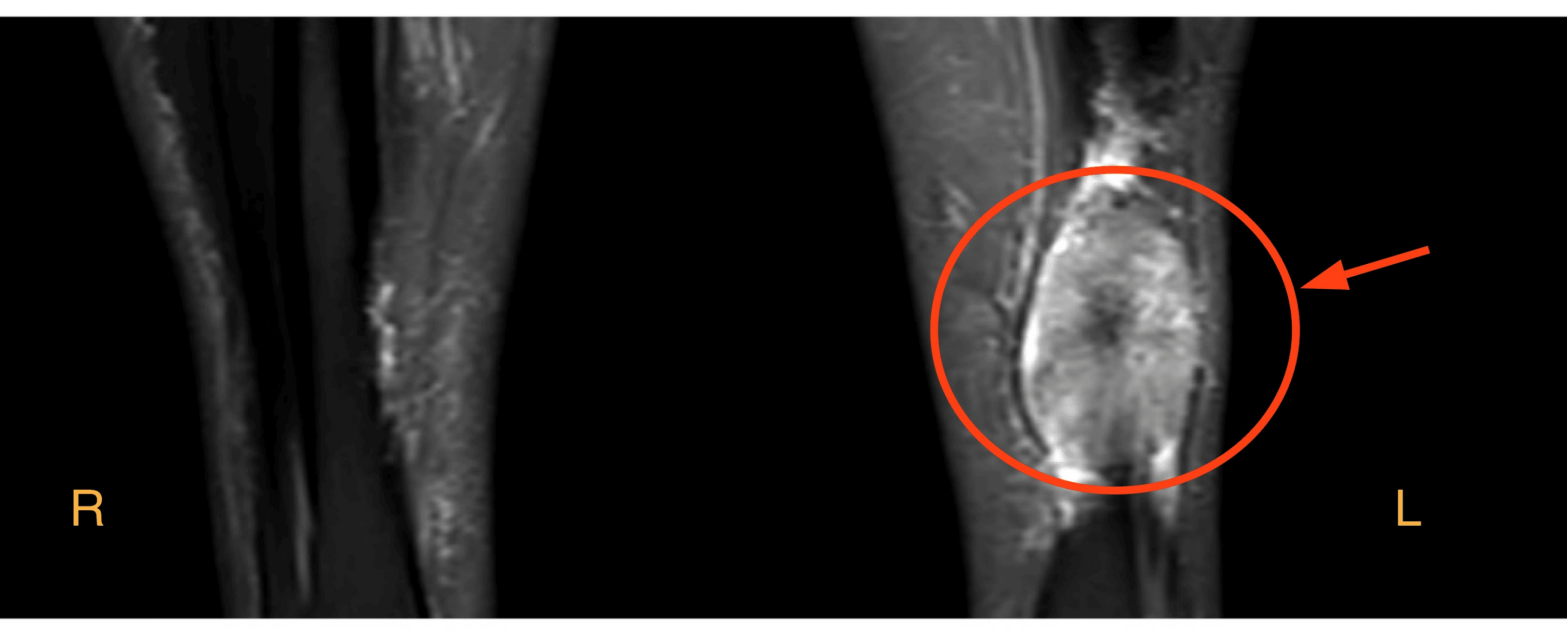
MRI of the legs There is a distal tibial well-demarcated soft tissue mass overlying the anteromedial tibia (arrowhead) associated with spiculated periosteal reaction (likely a superficial invasion). The mass contains a few internal calcification and demonstrates heterogeneous T1WI isointensity and heterogeneous T2 hyperintensity with heterogeneous postcontrast enhancement measuring 6.3 × 4.1 × 1.3 cm worrisome of underlying soft tissue sarcoma. T1WI: T1-weighted imaging; R: right; L: left
